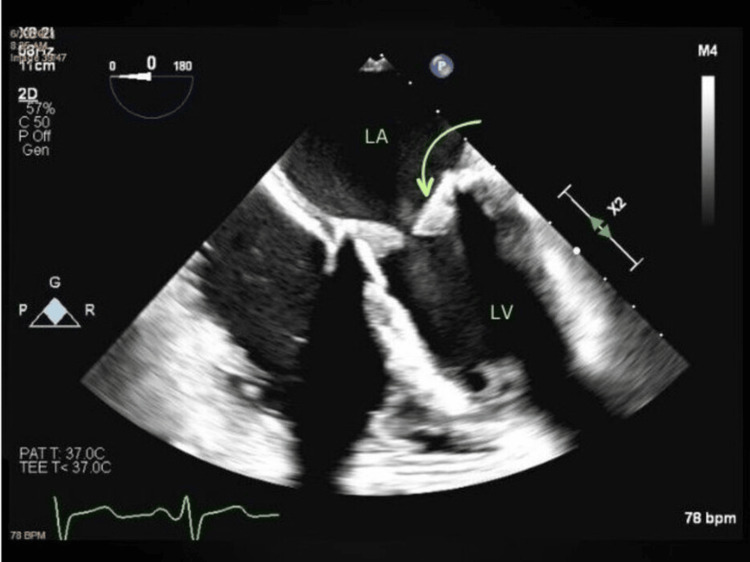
June 2023 TEE TEE from June 2023 showing a grossly thickened bioprosthetic mitral valve with echogenic material in the ventricular side of the leaflets (in green arrow) TEE: transesophageal echocardiogram; LA: left atrium; LV: left ventricle
